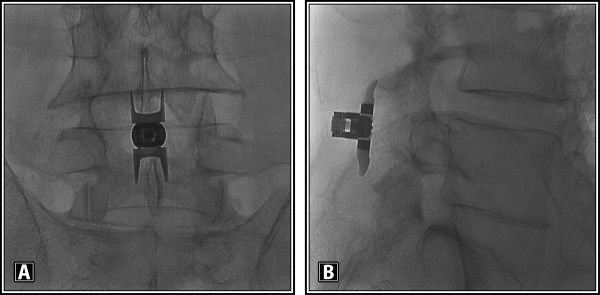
(A) A/P and (B) lateral radiographic image showing a properly placed
